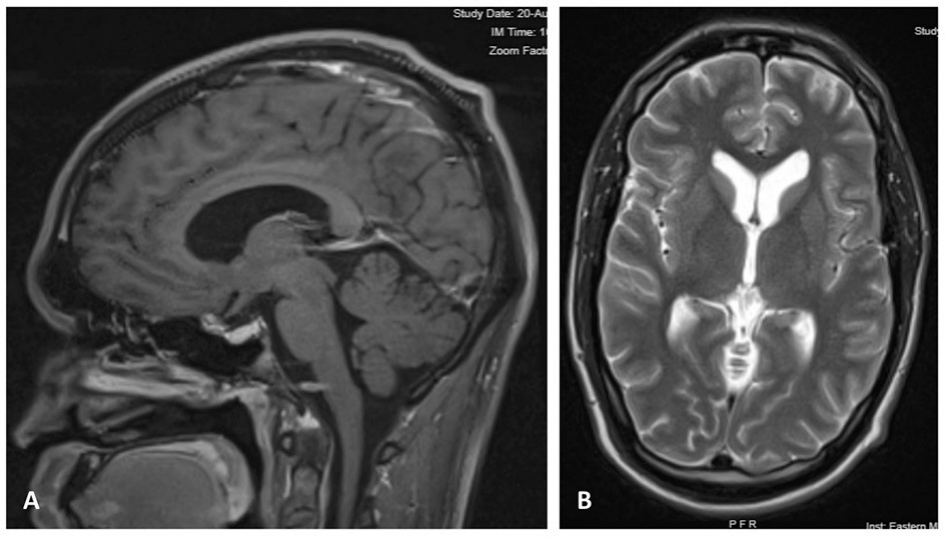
(A) Contrast-enhanced Sagittal T1 image 6 months after craniotomy, showing decompression of the sellar region with resolved mass effect over the optic pathway. (B) Axial T2 MRI at 6 months after surgery, showing normal size of ventricles and sulci.
